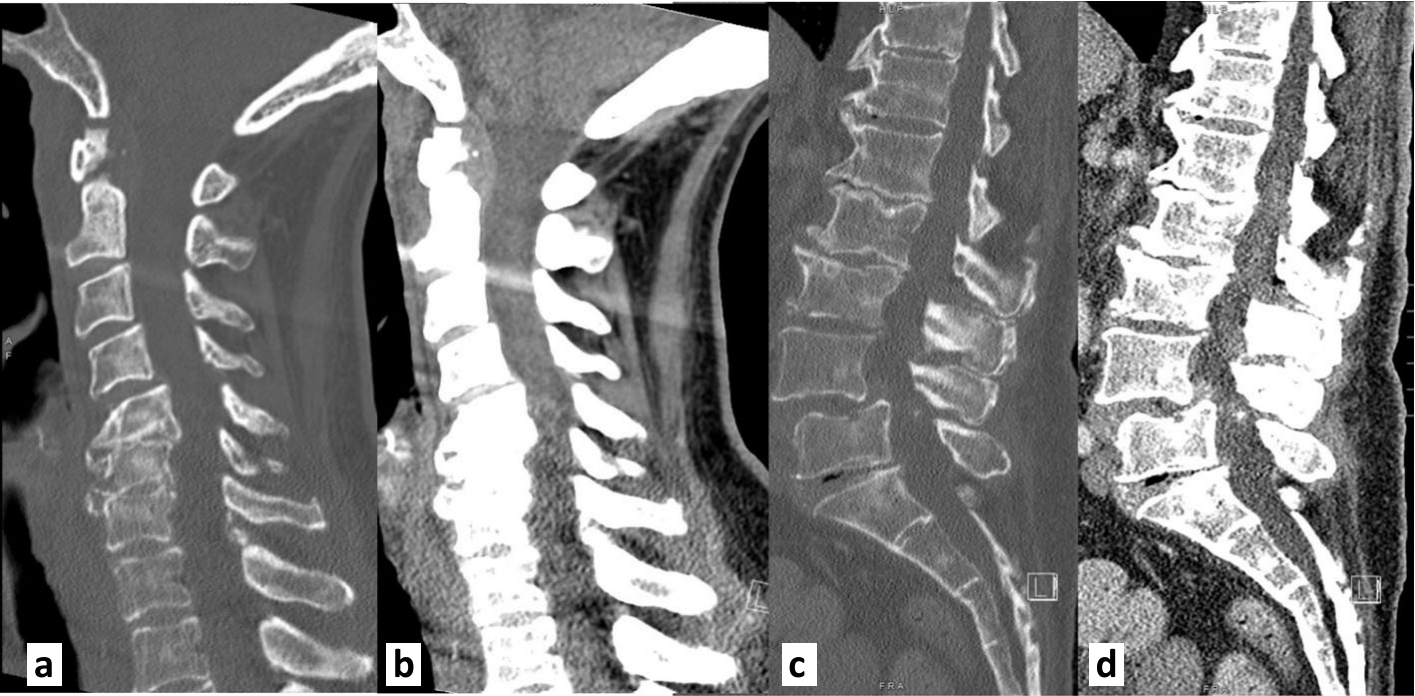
Computed tomography scan of cervical and lumbar spines. a-b Diffuse degenerative disc disease and spondylosis significantly at C5-C6 and C6-C7. Anterior offset of C4 on C5. c-d Degenerative disc disease and diffuse spondylotic changes at L4-L5, L5-S1 levels in lumbar spine along with disc space narrowing at all levels. Grade 1 anterolisthesis of L4 on L5
